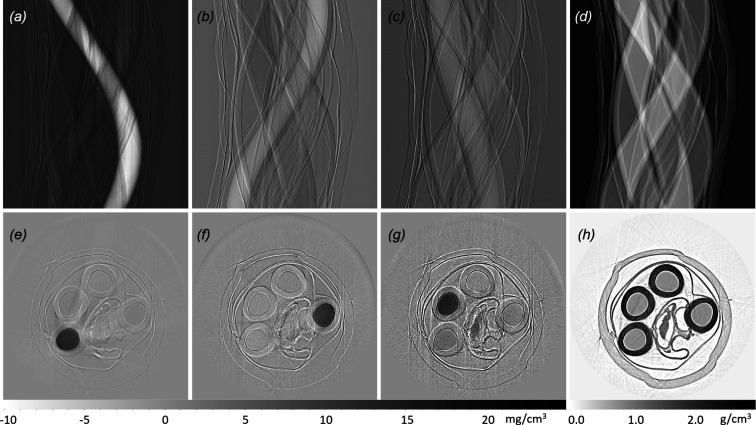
Sinograms of the distribution of (*a*) selenate, (*b*) selenite, (*c*) selenomethionine and (*d*) water. The CT reconstruction of the density distributions of (*e*) selenate, (*f*) selenite, (*g*) selenomethionine and (*h*) water. Every Se compound solution is prepared with 100 m*M* concentration. 7 mg cm^−3^ selenomethionine was detected in the seedpod of *Astragalus bisulcatus*, which is placed in the center of the sample holder. The gray scale bar on the bottom left is shown for the corresponding Se compounds concentrations in (*e*), (*f*) and (*g*), and the gray scale bar on the bottom right for (*h*).
